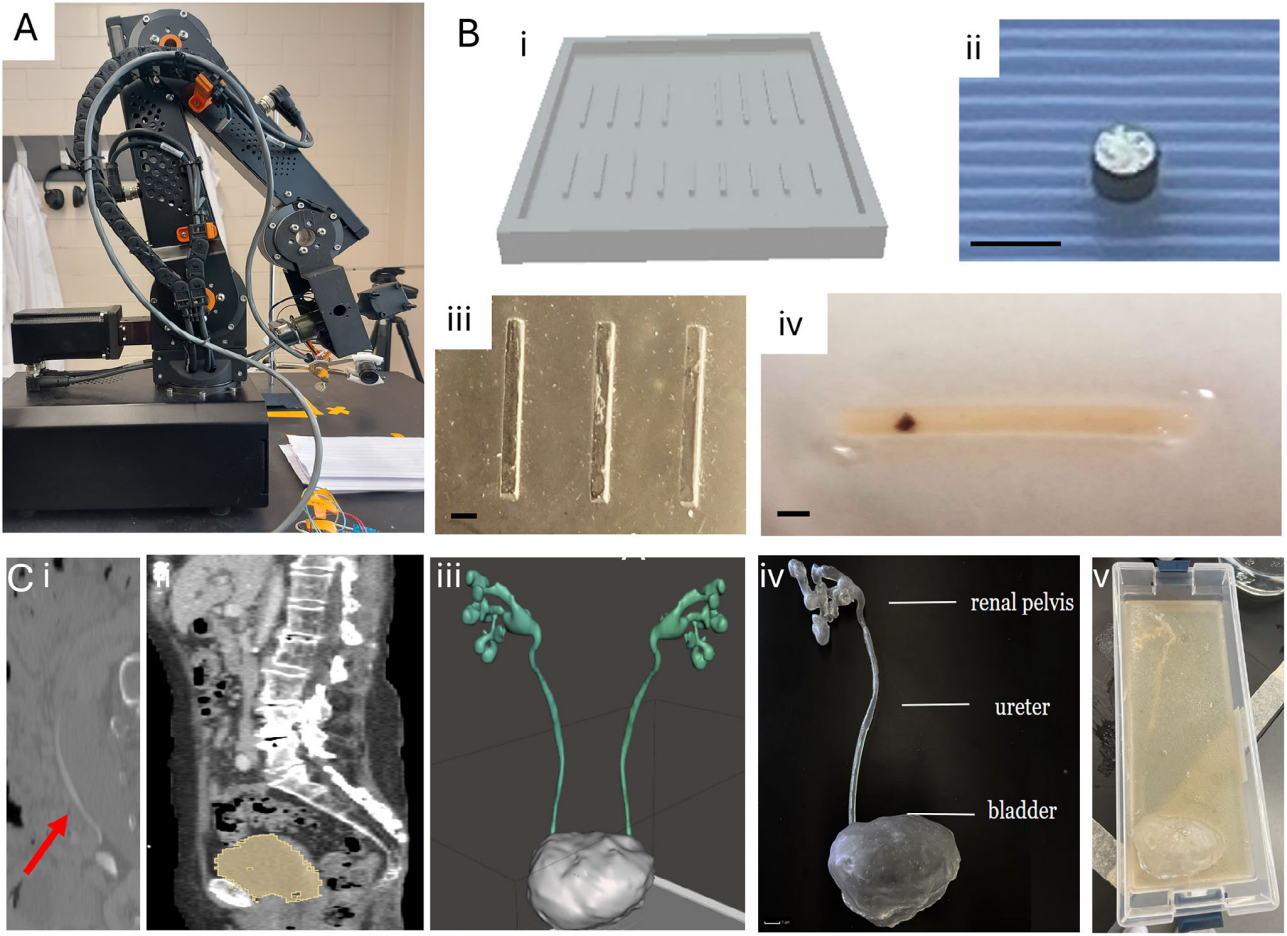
Magnetic actuation setup and fabrication of flexible filaments. A) Magnetic control system consisting of a 4 degree of freedom robot arm steering a DC motor that rotated a casing containing a permanent magnet. Bi) 3D printed negative mold cast for fabrication of gelatin filaments. Bii) 0.5–0.7 mm micromagnet. Biii) positive PDMS mold for UV crosslinking of gelatin methacrylate with embedded micromagnet Biv) resulting soft filament with embedded micromagnet and loaded enzyme urease. C) Fabrication of human anatomical urinary tract model by segmentation and selection of organ of interest from CT images of ureter (Ci, red arrow pointing at ureter) and bladder (Cii), used to reconstruct the urinary tract including bladder, ureter and renal pelvis (Ciii). (Civ) 3D printed transparent elastic model, (Cv) embedded for ultrasound imaging. Scale bars are 1 mm.
